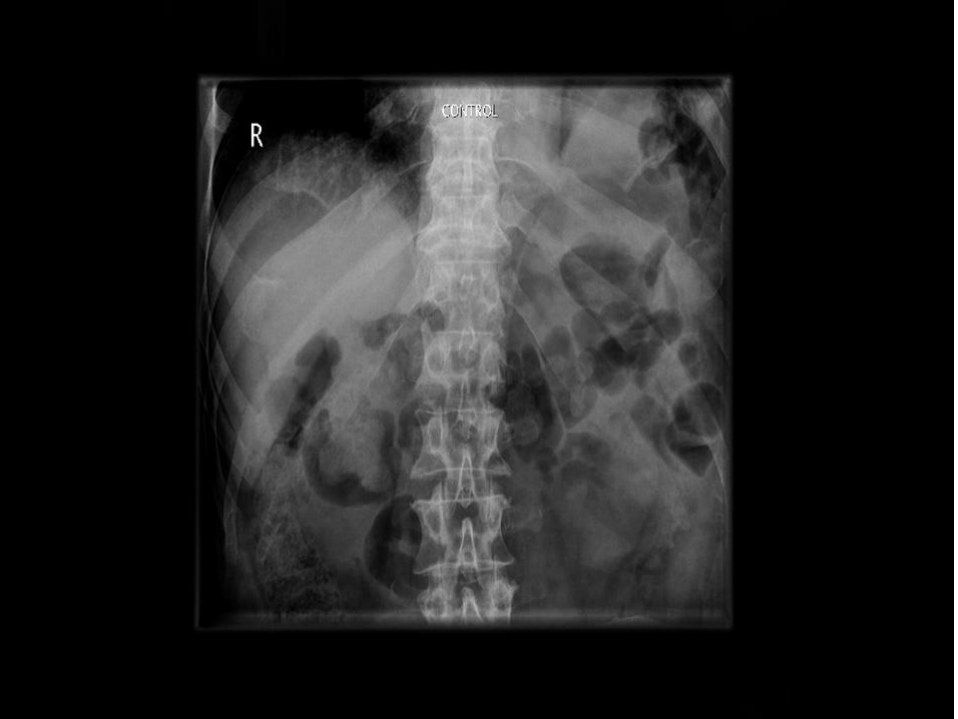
Plain abdominal x-ray on presentation. Note nonvisualization of the left hemidiaphragm and bowel gas at the left lung base.
